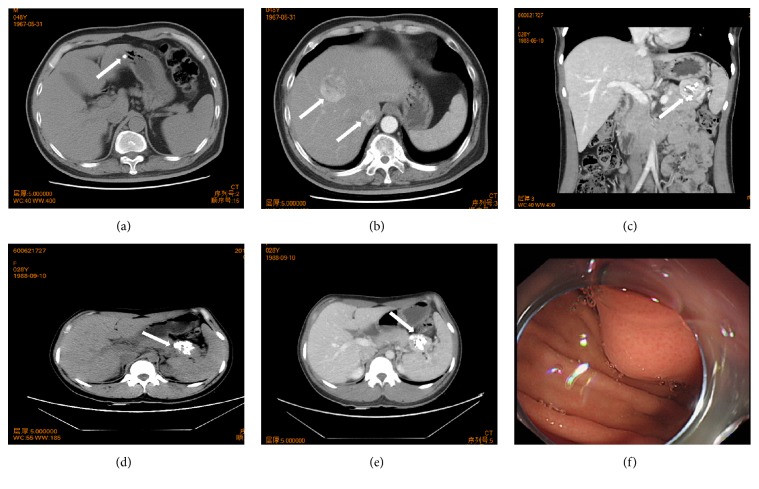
Imaging characteristics of CFT. Axial CT scan showed a soft-tissue density nodule (arrow) with centrally prominent calcification on the gastric wall (case 16) (a); meanwhile, the contrast-enhanced CT indicated two hepatic masses with mild enhancement (arrow; case 16) (b); the coronal CT scan demonstrated a round mass (arrow) with evident calcification in the upper-left abdominal cavity (case 19) (c); axial CT revealed a mass with calcification in the abdominal cavity in proximity to the tail of the pancreas (arrow; case 19) (d); contrast-enhanced CT indicated the well-defined mass mild enhancement peripherally (arrow; case 19) (e); gastroscopy showed a submucosal bulging mass (arrow) with overlying intact smooth mucosa (f).
